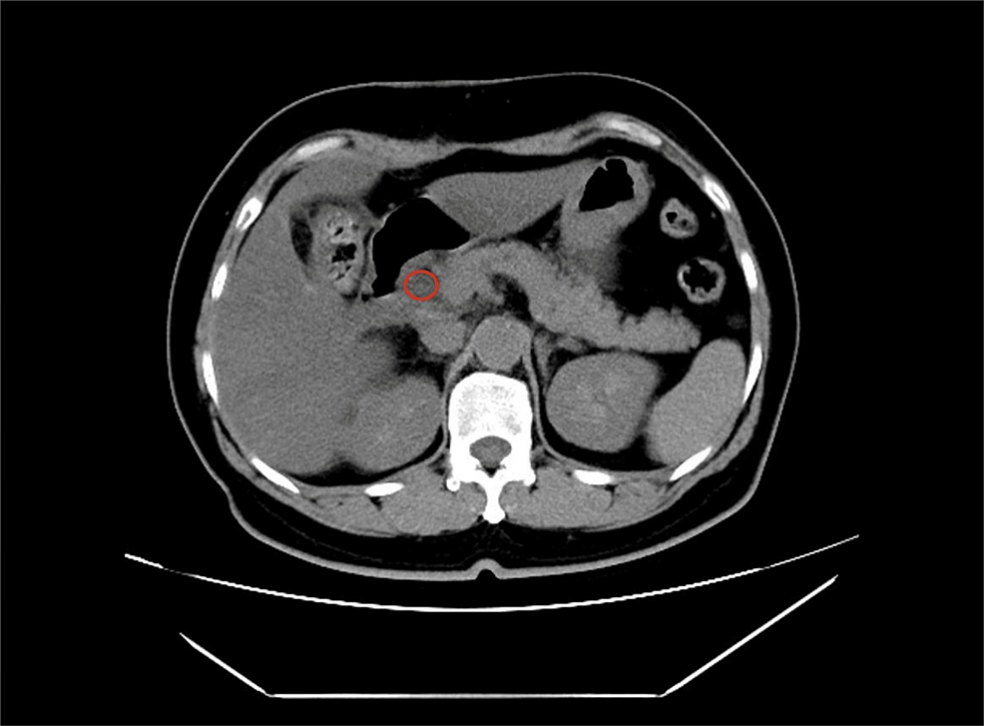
Postoperative CT scan of the upper abdomen showed a stone (marked in red) in the lower part of the common bile duct. CT: computed tomography
